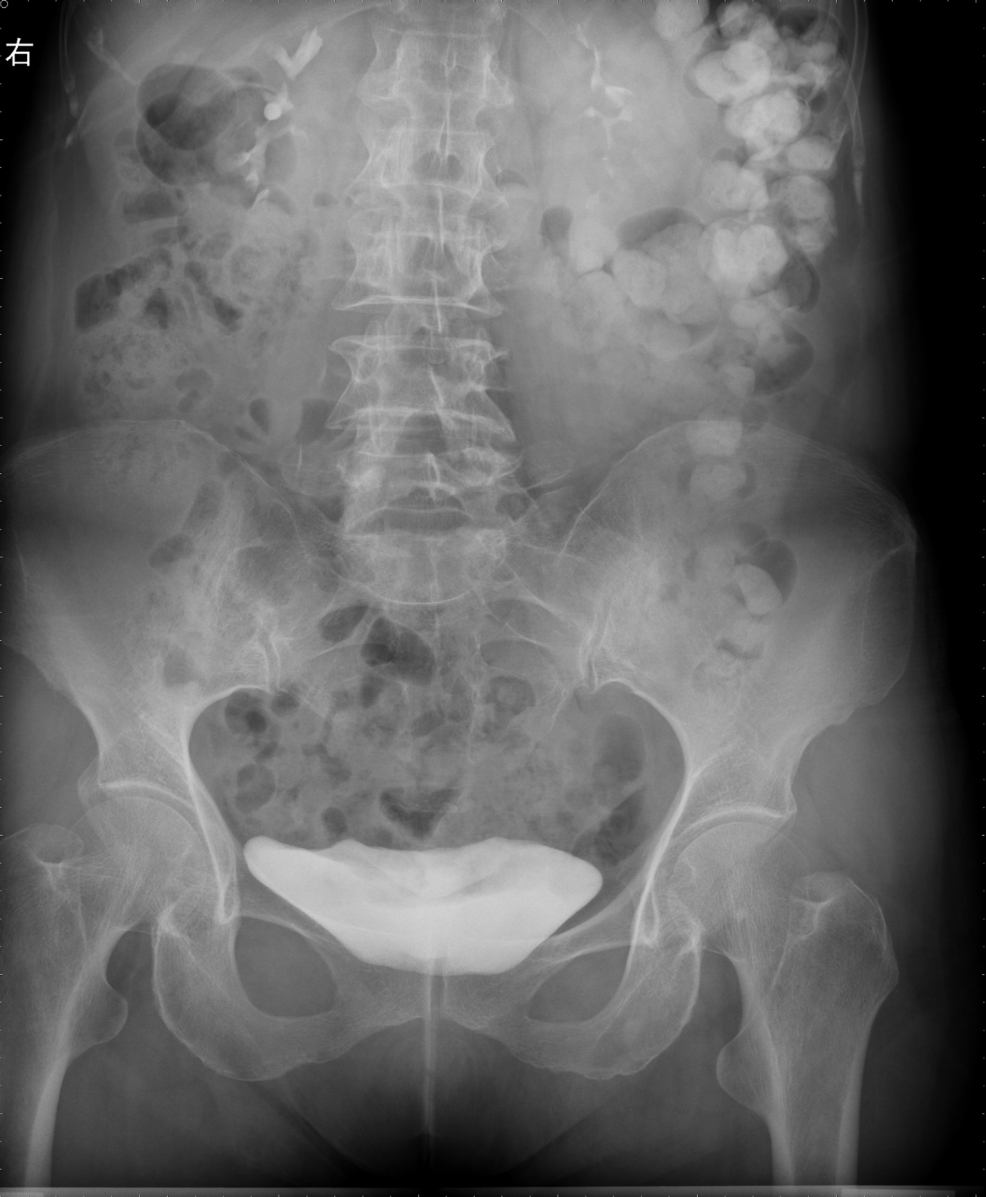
Abdominal X-ray imaging suggested that there was a dense shadow in the duodenal bulb with a length of about 2.7 cm, which might be considered a foreign body
